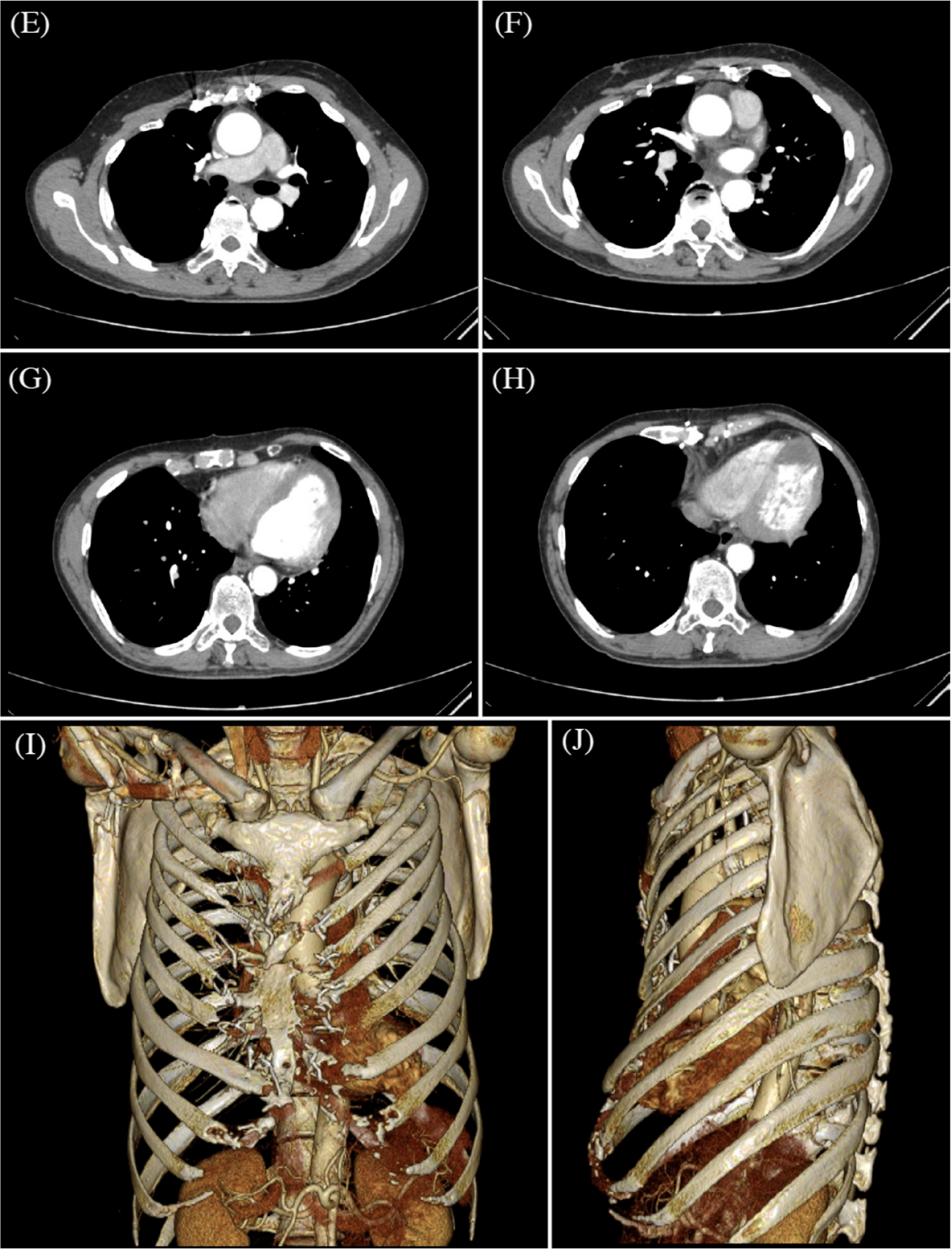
Preoperative axial (e–h) and three-dimensional (i, j) computed tomography shows bilateral occlusion of the internal thoracic arteries and a small fragile sternum after sternoturnover fixation
